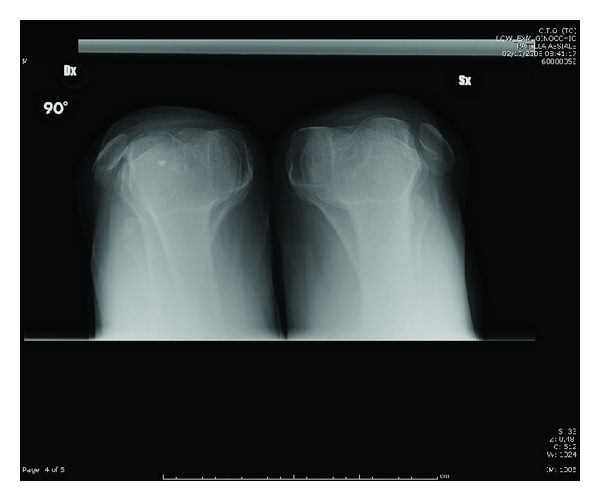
Skyline view roentgenograms, showing an increased deep of the trochlear groove and laterally dislocated patellae.
